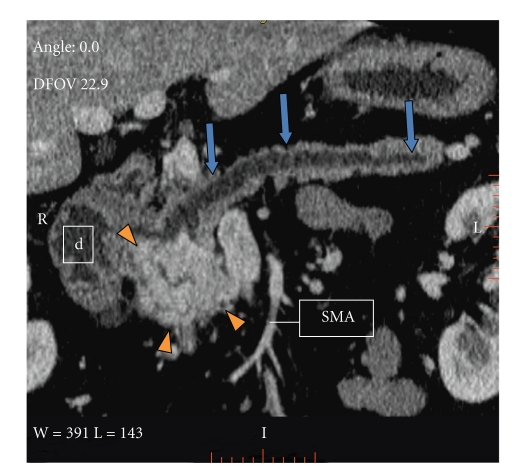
Contrast enhanced CT abdomen. Curved multiplanar reconstruction demonstrates an intimal relation of the retroperitoneal paraganglioma, and the proximal pancreas, duodenum (d), and IVC. Pancreatic body and tail show parenchymal atrophy and pancreatic ductal dilatation (arrows). SMA: superior mesenteric artery.
